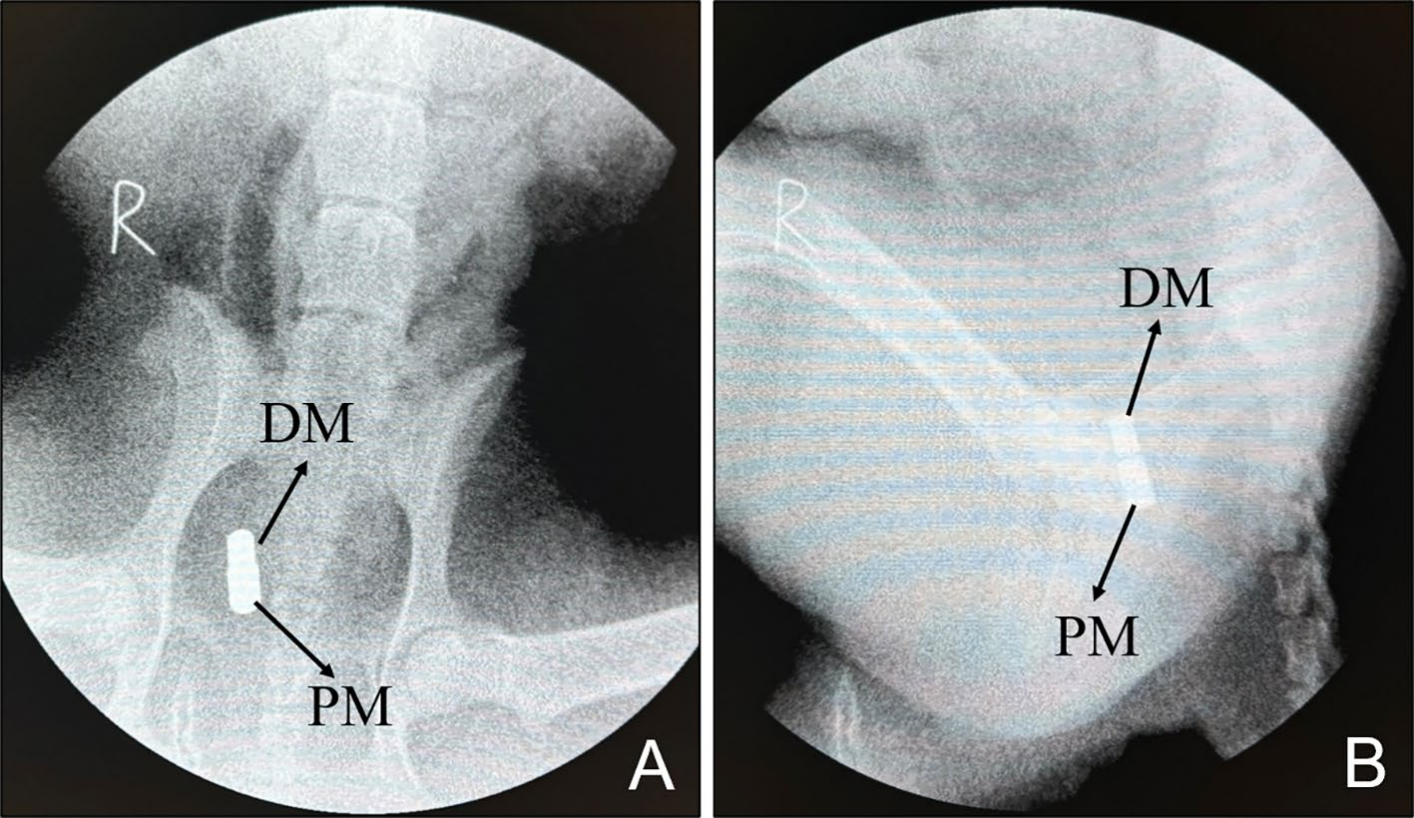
Postoperative X-ray examination. (A) Ventrodosal radiograph. (B) Lateral radiograph.
